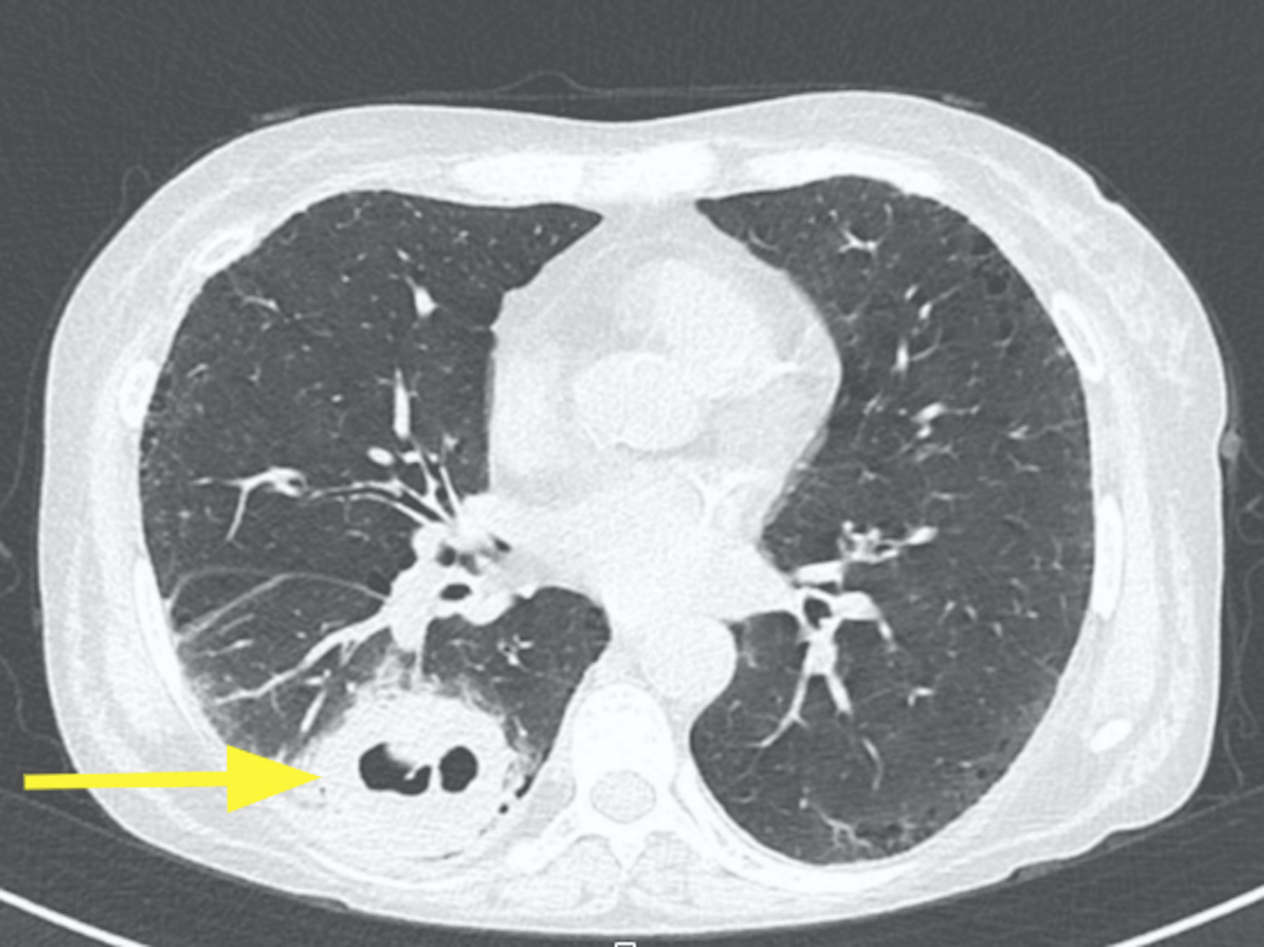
CT of the thorax revealing a mass in the basal segment of the lower right lobe (arrow) CT: computed tomography
